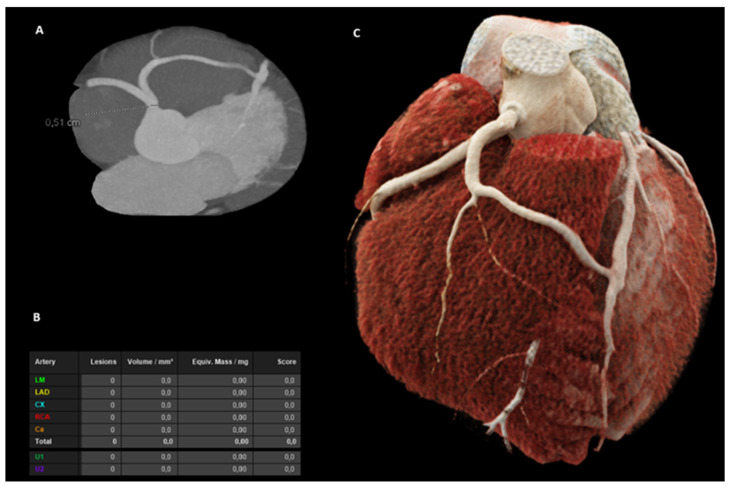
Coronary circulation was supplied via the right coronary artery (RCA), which arose normally from the aorta. The left anterior descending artery (LAD) originated from a wide branch of the RCA, while the LAD retrogradely supplied the left circumflex artery (LCX). After the Heart Team consultation, the patient was deemed qualified for coronary artery bypass grafting in the event of evidence of significant ischemia on single-photon emission computed tomography (SPECT). (A) Angio-CT visualization, (B) calcium score, and (C) 3D presentation.
